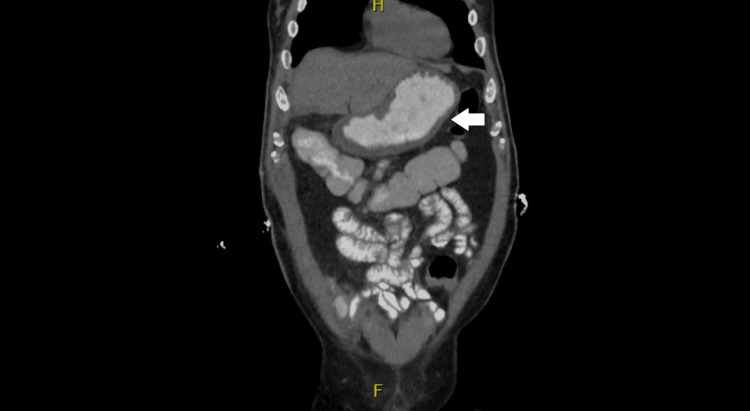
CT abdomen and pelvis showing resolution of gastric pneumatosis after six days of antibiotics and supportive care
